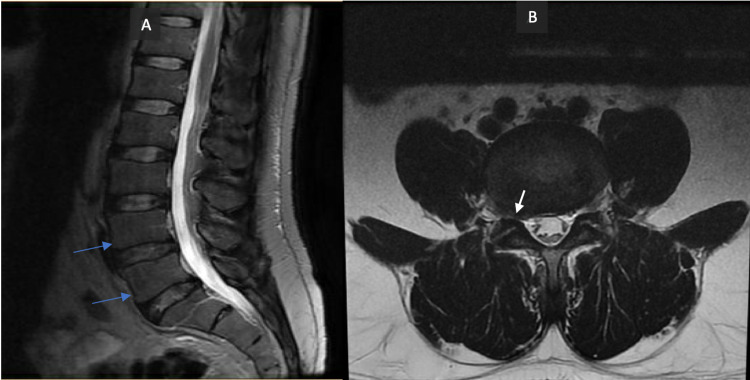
MRI of the lumbar spine (A): mild multilevel degenerative changes (blue arrow) on the sagittal plane and (B) complex asymmetric to the right with the mild facet arthropathy and ligamentum flavum hypertrophy causing moderate right neural foraminal stenosis (white arrow); mild to moderate left neural foraminal stenosis on the axial plane MRI: magnetic resonance imaging
